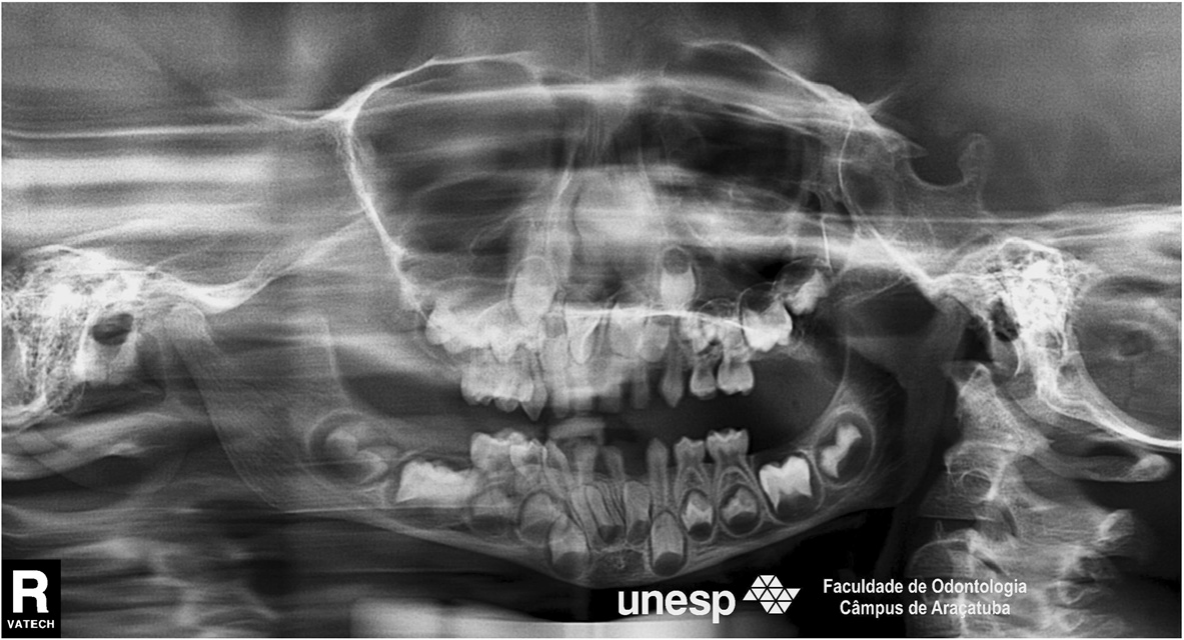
Orthopantomograph showing absence of a mandibular permanent incisor.
